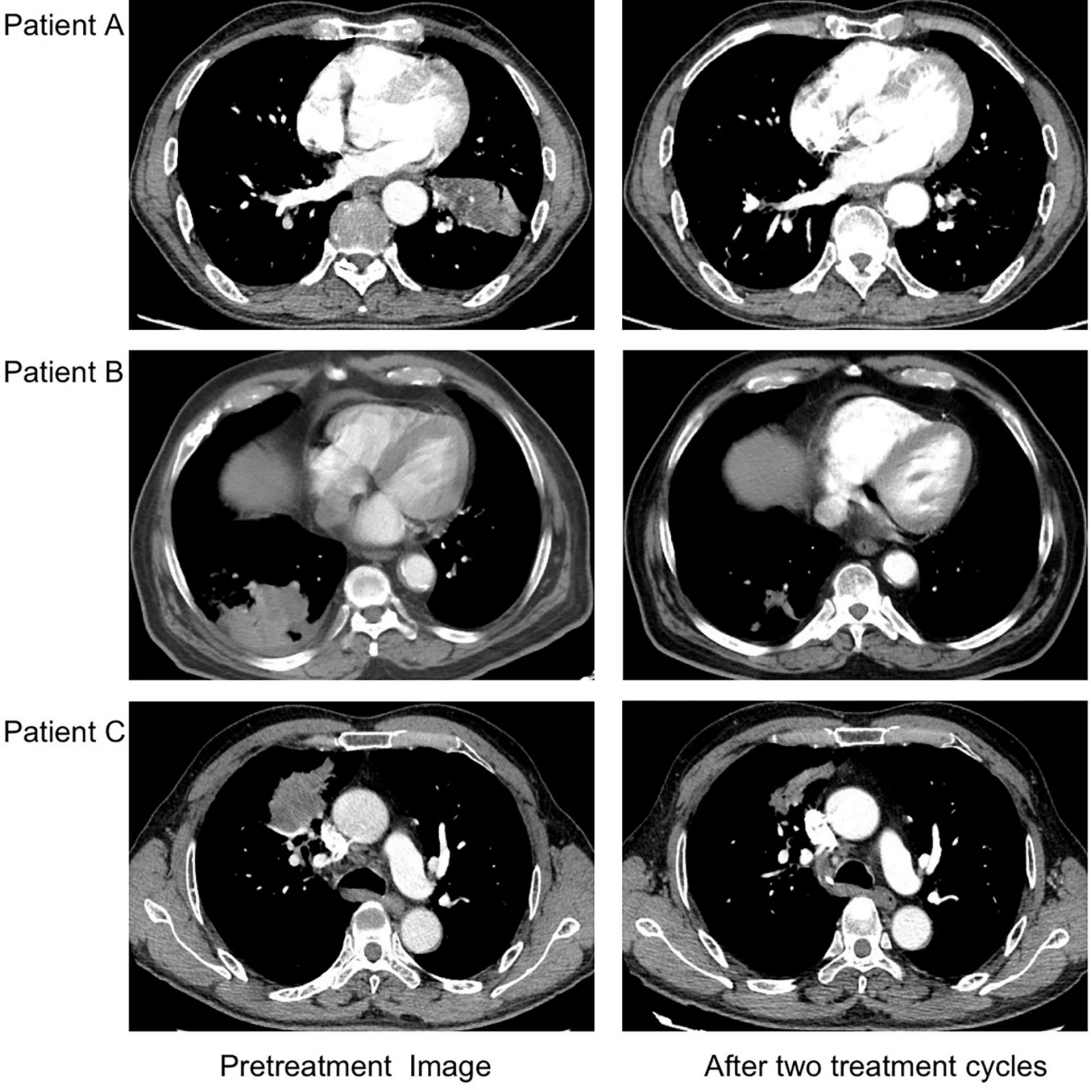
CT images of lesions before and after two cycles of neoadjuvant camrelizumab plus chemotherapy. After two doses of camrelizumab plus chemotherapy, the lesions of three patients were significantly reduced.
